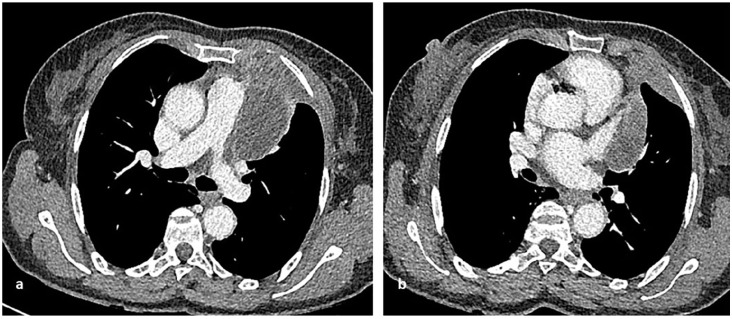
Preoperative computed tomography shows a left anterior mediastinal mass involving the main pulmonary artery, the proximal left pulmonary artery, and the left superior pulmonary vein but not the inferior. There is also involvement of the pericardium at the level of the left atrial appendage and of the left anterior para mediastinal thoracic wall. The mass does not extend inferiorly or posteriorly to the left hilum.
